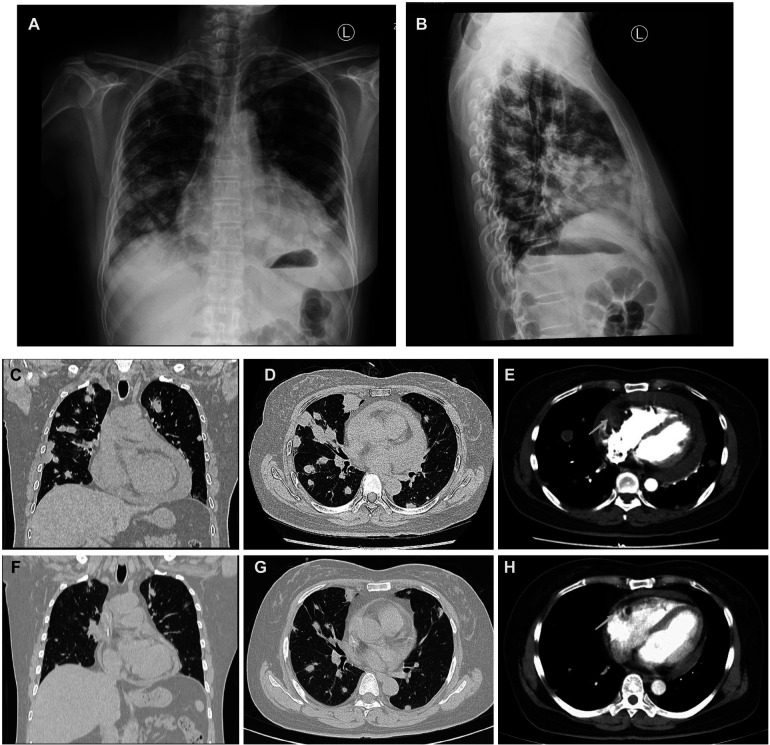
Comparison of lung metastases and cardiac mass before and after chemotherapy of Case2. (A,B) Frontal and lateral Chest x-ray demonstrated extensive pulmonary lesions. (C) Chest CT at coronal view and (D) axial view revealed severe lung metastases. (E) Contrast-enhanced Chest CT reveals a mass lesion (arrow) along the superior and posterior right atrial wall without enhancement. After 5 courses of chemotherapy, the number of lung metastases (F,G) was less than before (C,D), and the lateral wall of the RA (H) has no occupied (arrow) compared to earlier (E).
